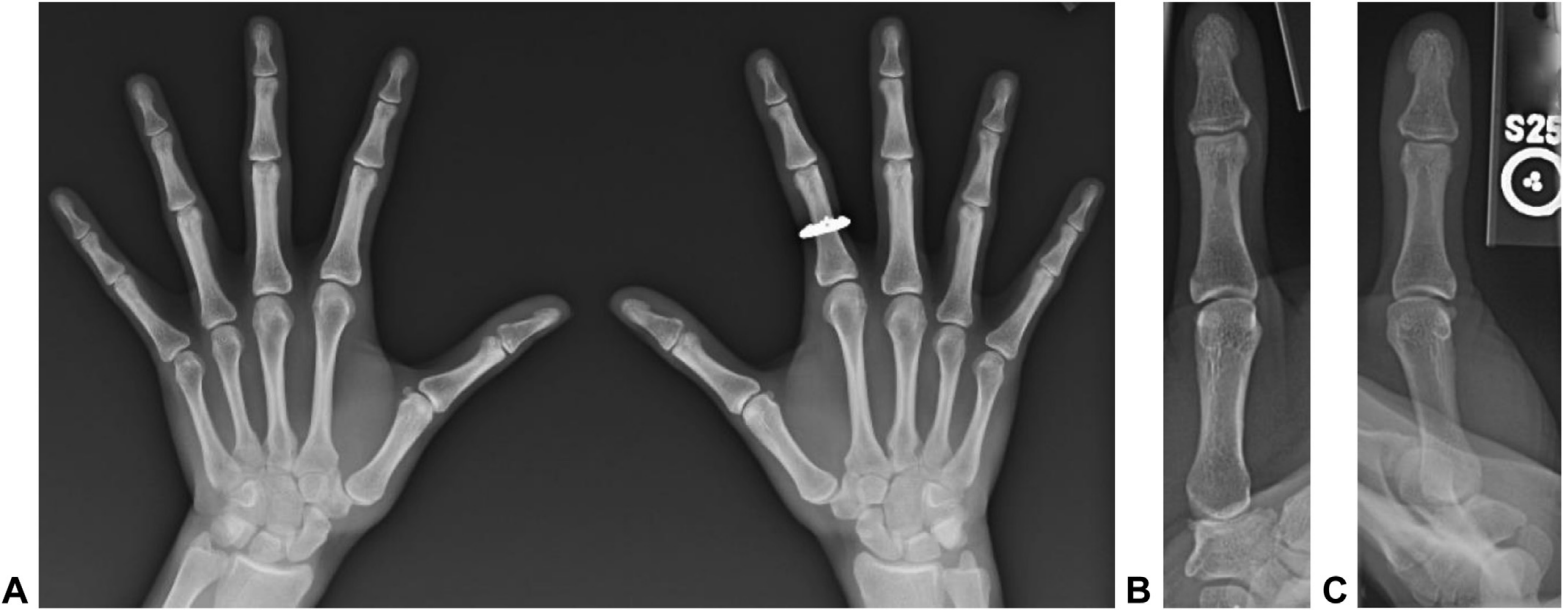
A Presenting posteroanterior bilateral hand radiographs left evidence of left thumb CMC radial subluxation with a steep trapezial articular slope. B and C Dedicated posteroanterior and oblique left thumb radiographs with evidence of mild CMC joint space narrowing and osteophytosis.
